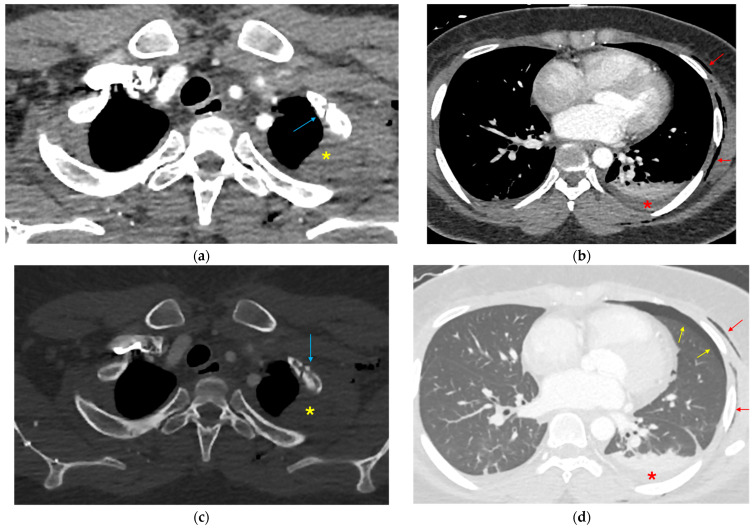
Multiple indirect signs in a patient with ATAI. (a,b) Axial soft tissue, (c) bone and (d) lung window CTA images show indirect signs of aortic injury, including left-sided first rib fracture (blue arrows), with adjacent pleural/extrapleural hematoma (yellow asterisks), left hemothorax (red asterisks), pneumothorax (yellow arrows) and subcutaneous emphysema (red arrows).
